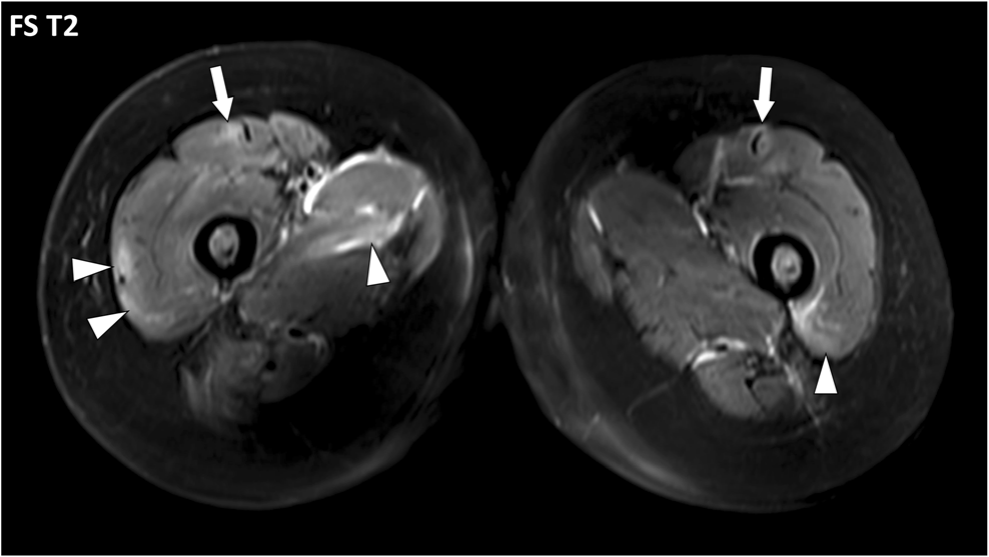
Axial fat-suppressed T2-weighted image of the thighs of a 49-year-old female with systemic lupus erythematosus, antiphospholipid syndrome and normal CPK. Multiple foci of increased signal intensity of the fascial system are depicted and they are all located at the periphery of the muscles near the deep fasciae (arrowheads) or near the fibro-muscular interfaces (arrows) suggesting involvement of the epimysium
